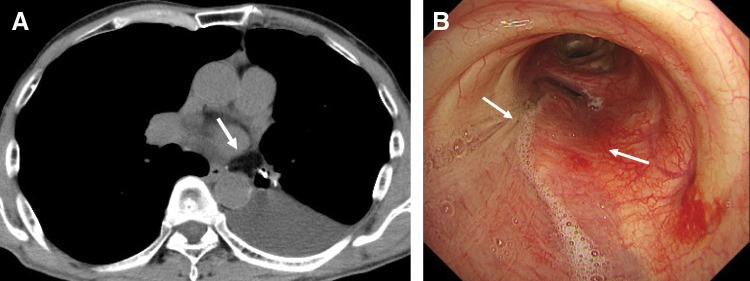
Postoperative imaging on day 6. (A) Chest CT shows the free fat pad adherent along the right-to-dorsal aspect of the left main bronchus and the second carina (arrow). (B) Bronchoscopy reveals loss of longitudinal folds at the previously thinned membranous portion of the left main bronchus, with the underlying fat pad visible through the membrane (arrows).
